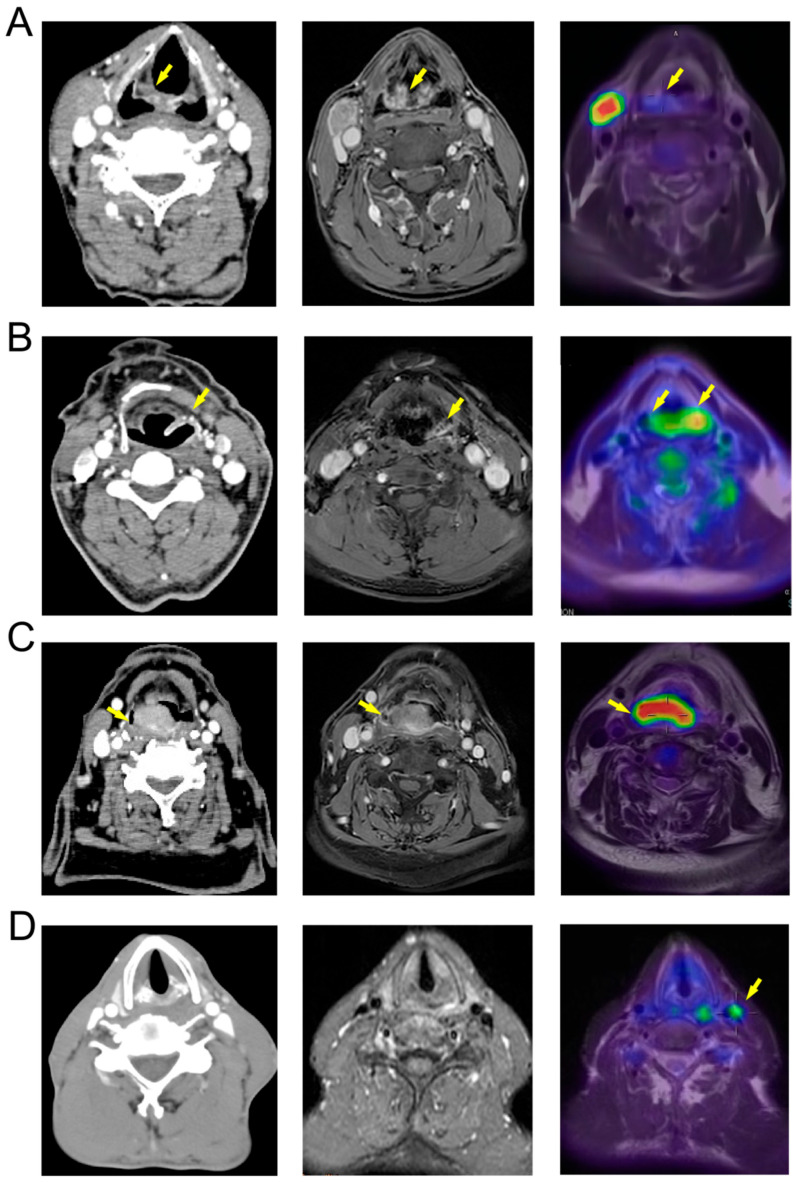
Comparison of CT, MR, and PET/MR images of HPC. (A) The primary lesion (yellow arrow) is located on the medial wall of the right piriform sinus (T1) and is difficult to distinguish in CT and MR images, but shows increased metabolism in PET/MR; (B) The primary lesion (yellow arrow) is located on the medial, lateral, and posterior walls of the left hypopharynx (T3), appearing more localized in CT and MR images, while PET/MR shows a high-metabolism lesion extending beyond the midline, consistent with intraoperative findings; (C) The primary lesion (yellow arrow) is located on the posterior wall of the pharynx (T3), with unclear boundaries in CT and MR images and suspected involvement of the prevertebral fascia, while PET/MR indicates that the tumor does not breach the prevertebral fascia; (D) The patient’s primary lesion is located in the left piriform sinus (T3), with no obvious positive lymph nodes shown in CT and MR, but PET/MR indicates high metabolism in the left cervical sheath lymph nodes (yellow arrow), with postoperative pathology indicating positivity.
